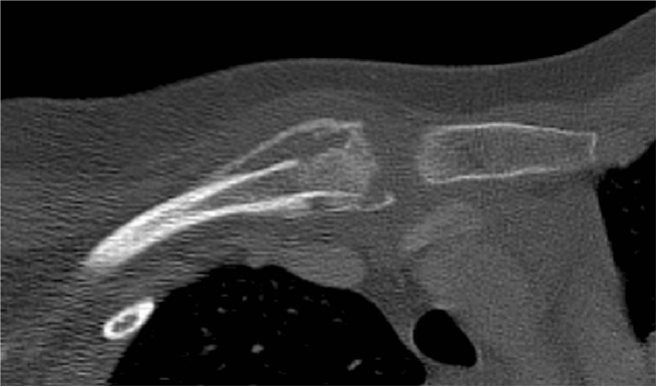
Computed tomography scan of the clavicle with cortical osteolysis and bowl-shaped periosteal hyperostosis.
